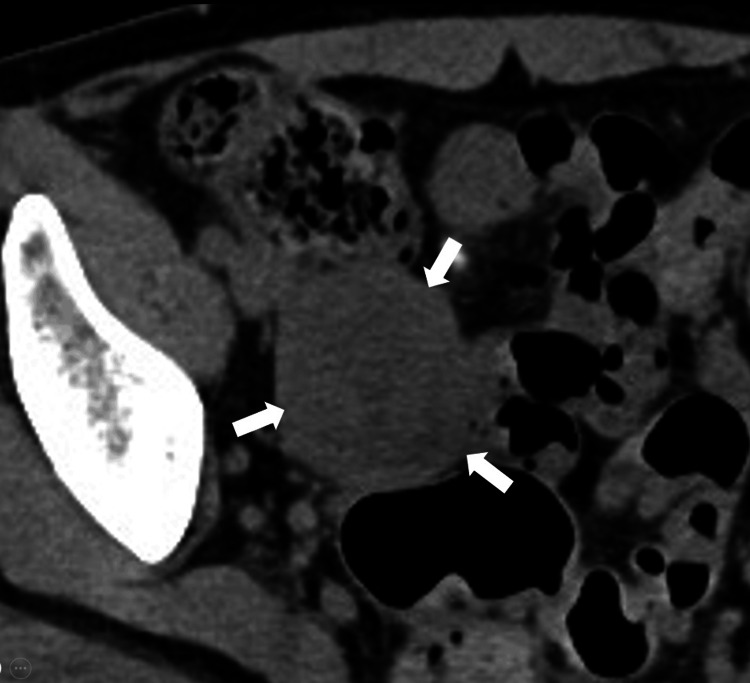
Unenhanced computed tomography (CT) image of the right adnexal mass. Unenhanced CT for systemic screening showed a solid mass in the right adnexa with 4.0 × 3.6 cm diameter and low density in comparison to the skeletal muscle.
